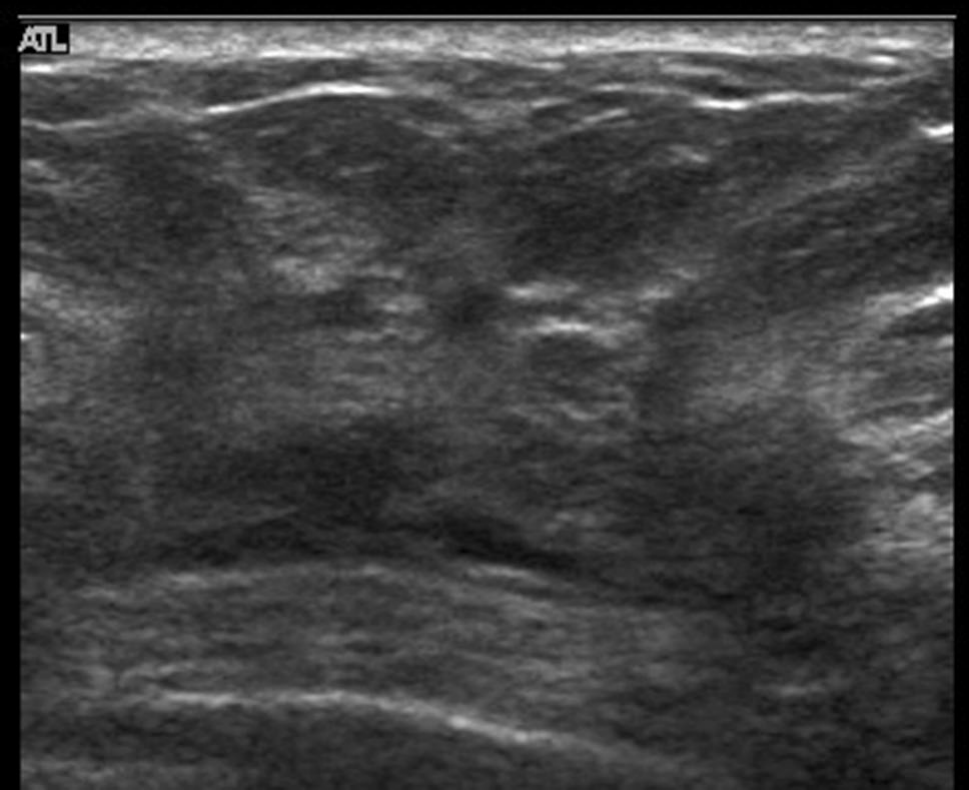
Case of invasive lobular carcinoma. Ultrasound shows the architectural distortion, but it is somewhat difficult to evaluate the architectural distortion
